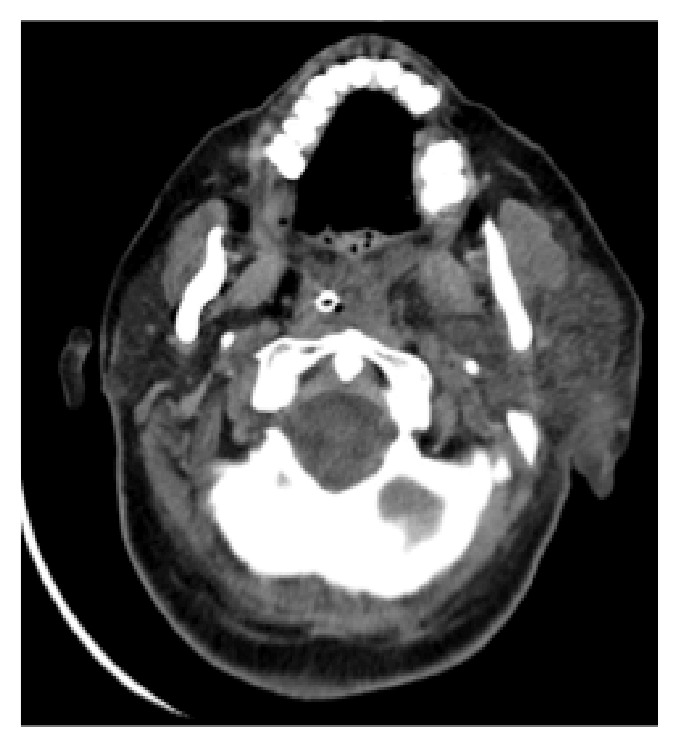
Head CT demonstrating left parotiditis.
